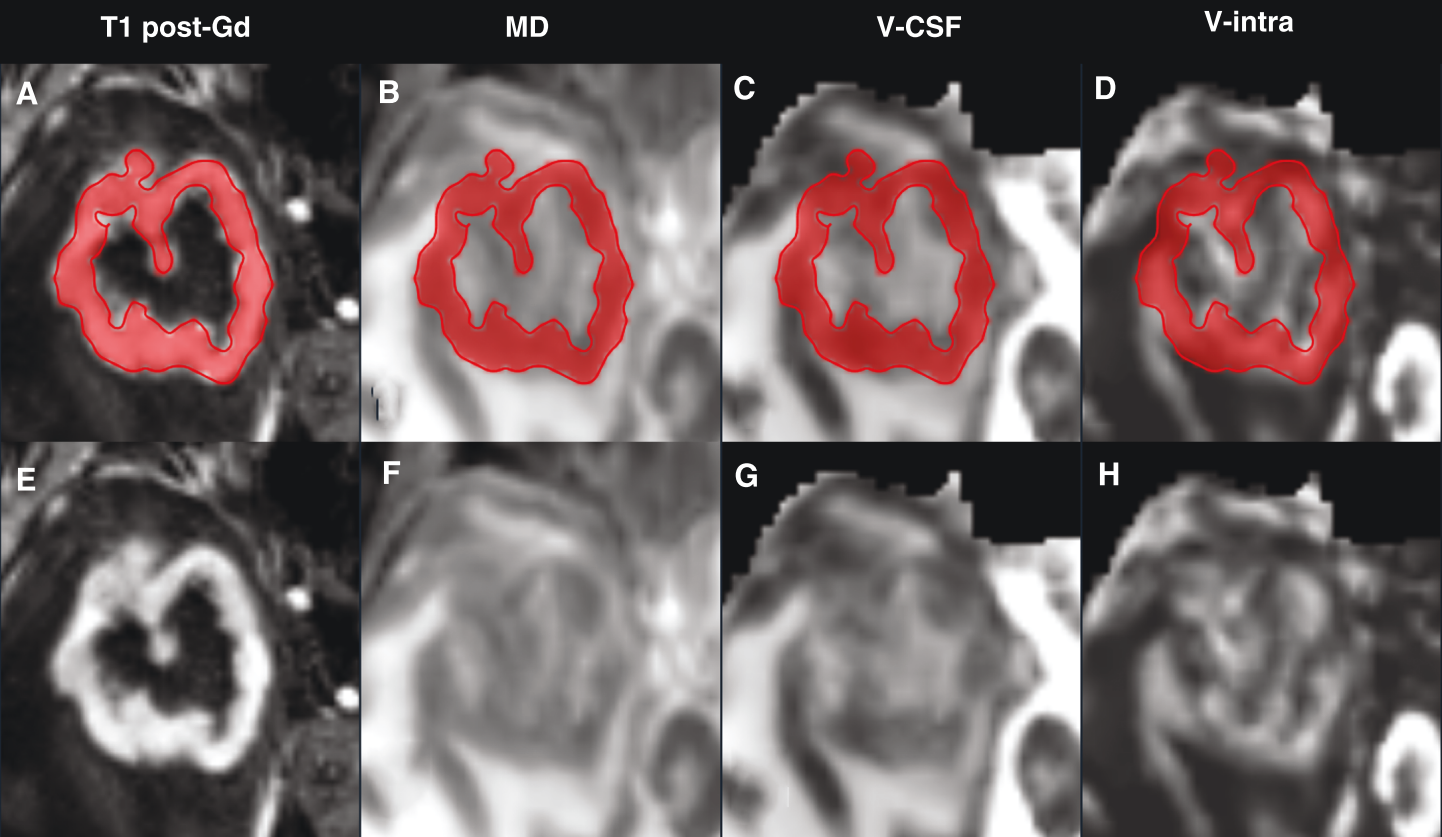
Presurgical (3T) MR imaging in a patient with an atypical (central necrotic) right temporal PCNSL. Representative axial images are shown in the upper row both with (A–D) and without (E–G) the corresponding ROI of the contrast-enhancing tumor component based on (A). Gd indicates Gadoteridol.
